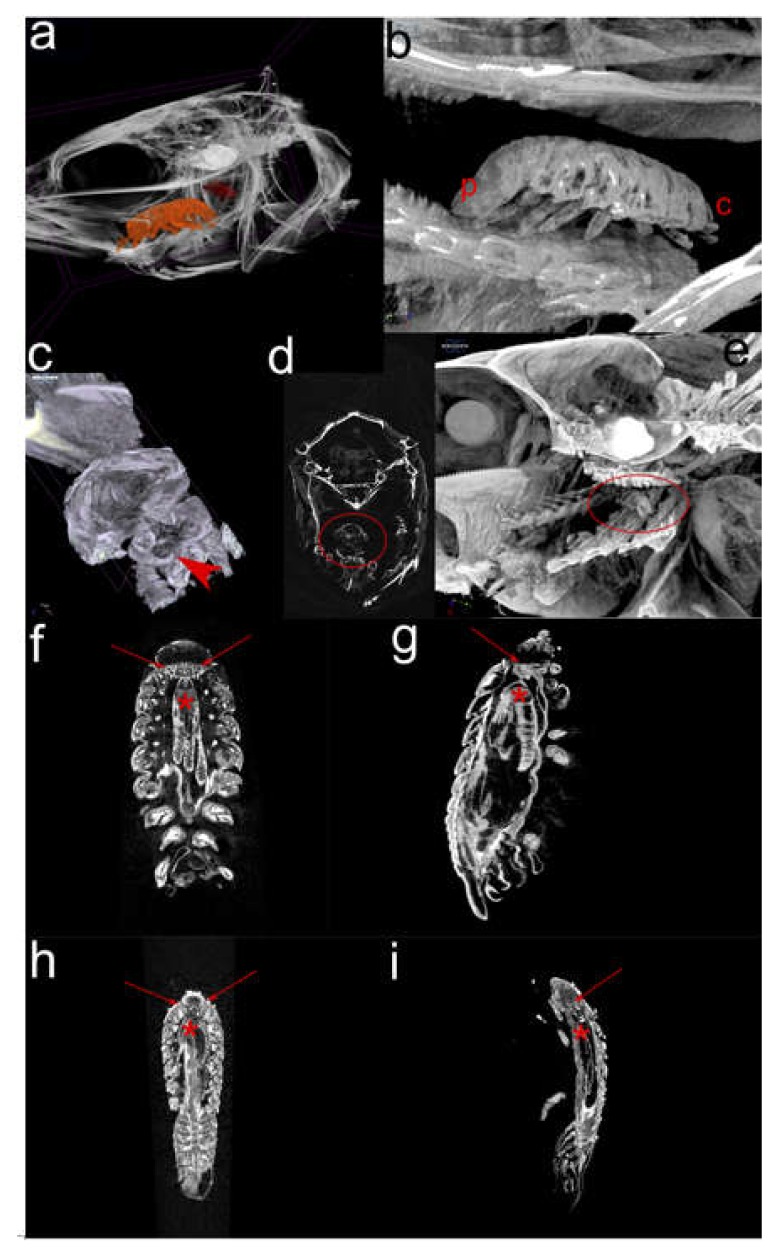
Selected renderings of 2 and 3D micro-computational tomography (µ-CT) of adult female and male *Ceratothoa oestroides* parasitising European sea bass (*Dicentrarchus labrax*) buccal cavity: (a) *In situ* 3D visualisation of female (orange) and male (red) positions; (b) Magnified 3D rendering of female showing attachment of the pereopods (p: pleotelson; c: cephalon); (c) 3D rendering of posterior paired pereopods piercing deep into lower limbs of gill arches (arrowhead); (d) Frontal 2D visualisation of female showing no direct contact between her mouthparts and host tissues (encircled); (e) Lateral 3D visualisation of male position (encircled); (f) Longitudinal 2D visualisation of female showing gland-like structures in the cephalon region (arrows) and mid-gut diverticula (asterisk), and (g) same, but in the lateral plane; (h) Longitudinal 2D visualisation of male showing no gland-like structures in the cephalon region (arrows) and mid-gut diverticula (asterisk), and (i) same, but in the lateral plane. Note that the software for figure construction dose not enable scale bar insertion, therefore, refer to other figures for the dimensions.
